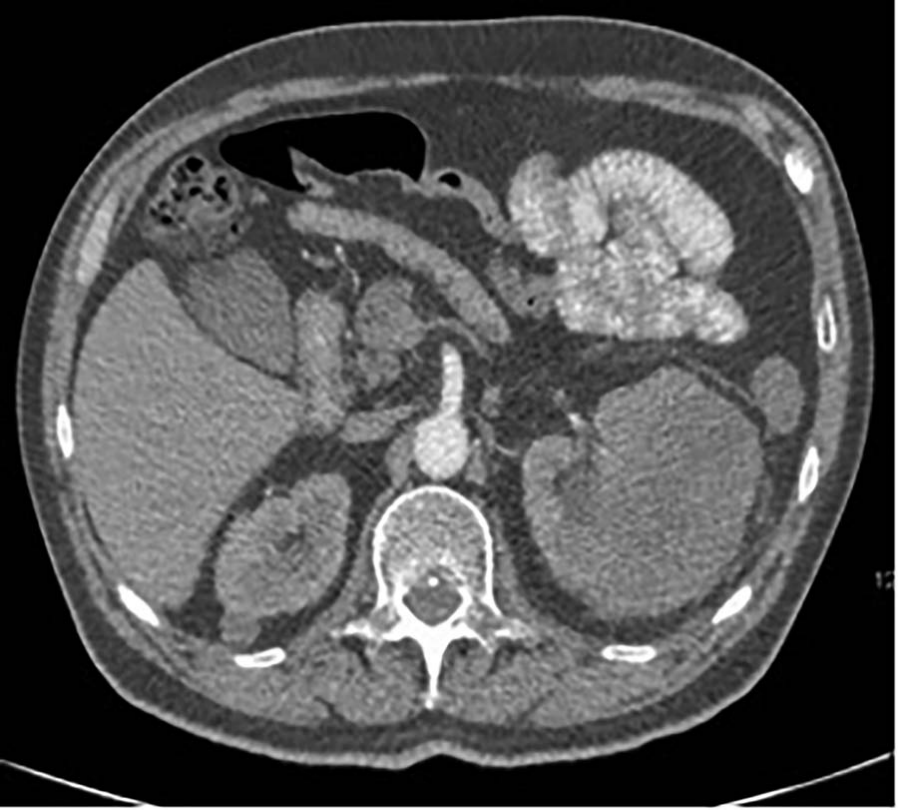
Axial CT showing a large 12-cm left renal heterogenous mass; no renal obstruction, hydronephrosis or calculus; the mass abuts the lower edge of the spleen.
